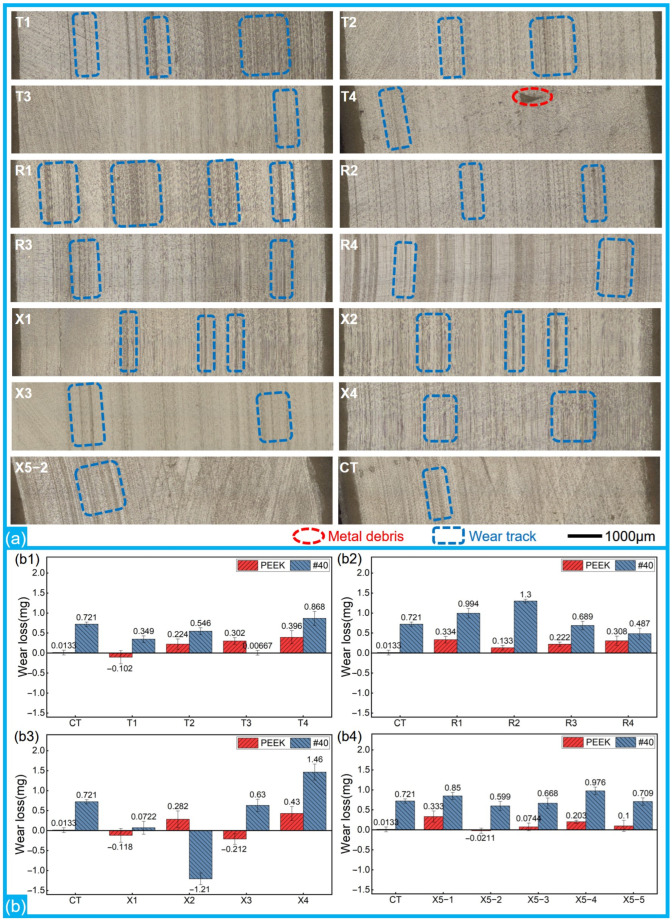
Representative wear morphologies and wear losses: (a) wear morphologies of the PEEK rings after ultrasonic washing; (b) wear losses of the PEEK rings and 1040 steel discs: (b1) wear losses of T1–T4; (b2) wear losses of R1–R4; (b3) wear losses of X1–X4; (b4) wear losses of X5-1~X5-5.
